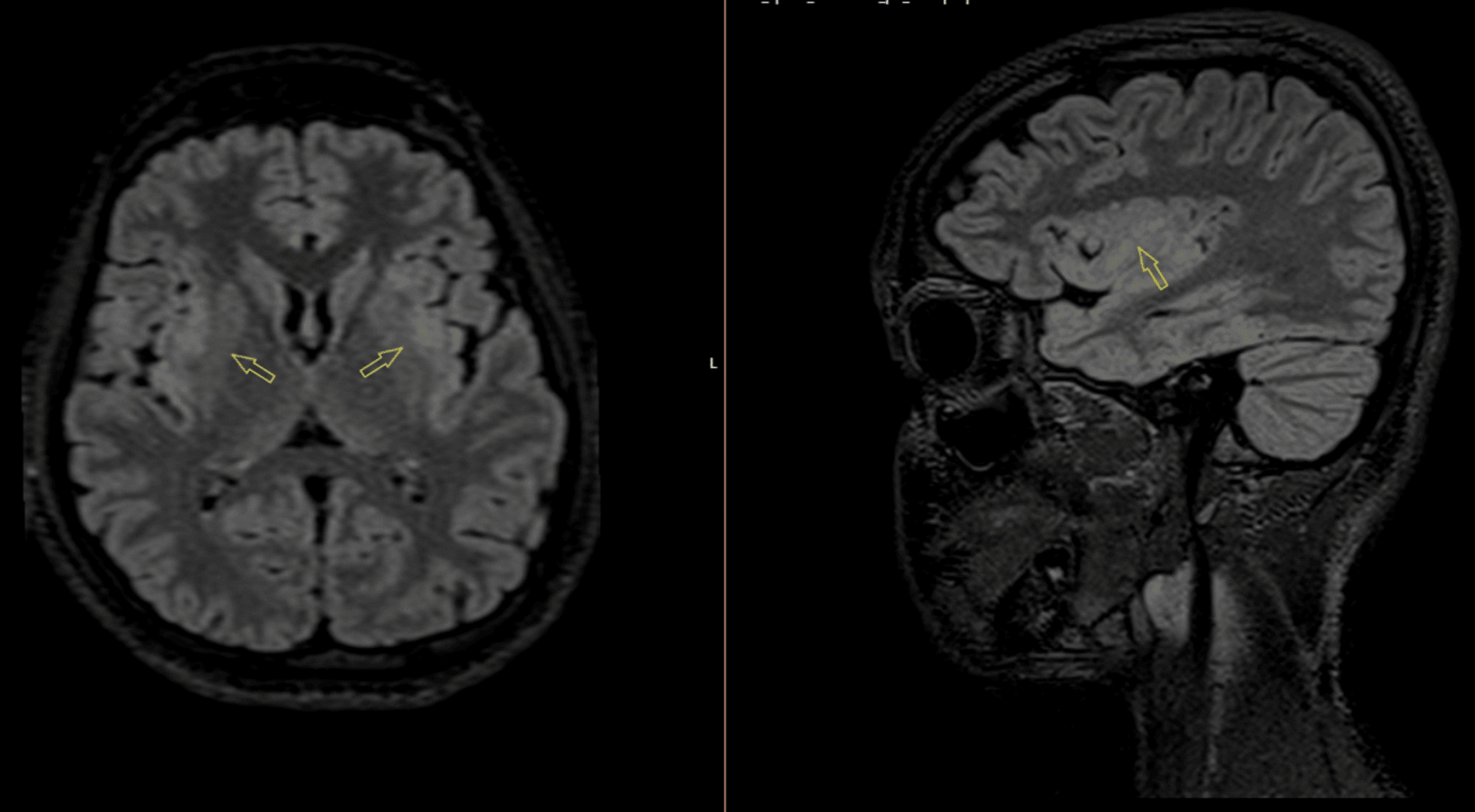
Axial fluid-attenuated inversion recovery (FLAIR) MRI showing swelling (edema) in both insular regions of the brain.
